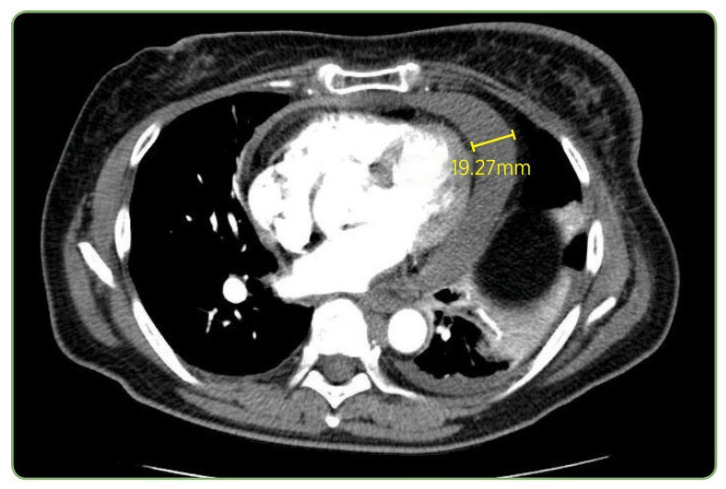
Representative CT pulmonary embolus study from the test set showing pericardial effusion measuring 19.27 mm.
